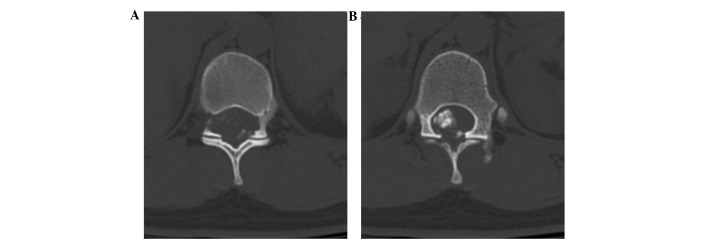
Axial computed tomography scan slice at thoracic level 11, showing extension of the neural foramina on the right, with (A) foraminal enlargement and (B) an isodense mass with calcification.
